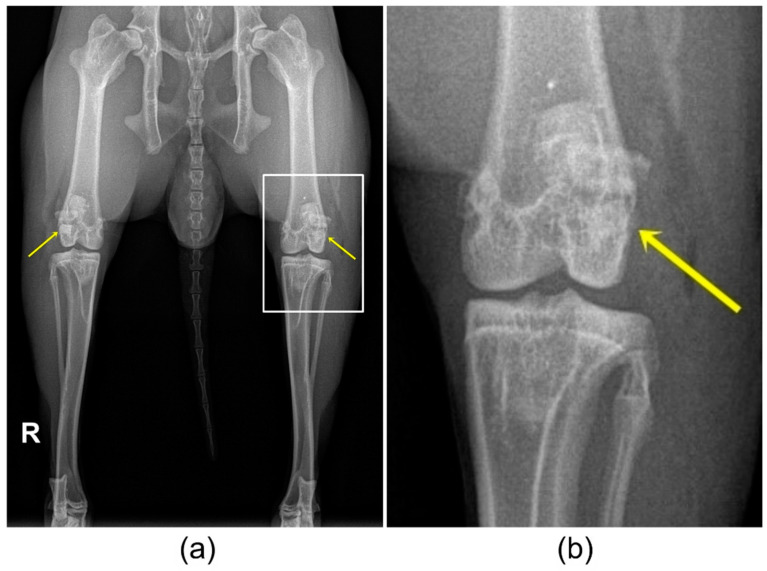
(a) Ventrodorsal (VD) X-ray view of the hind limbs directly after surgery with TCP implanted in the right and Mg225 in the left femoral condyle. (b) Magnification of the left knee joint with implanted Mg225 scaffold.
